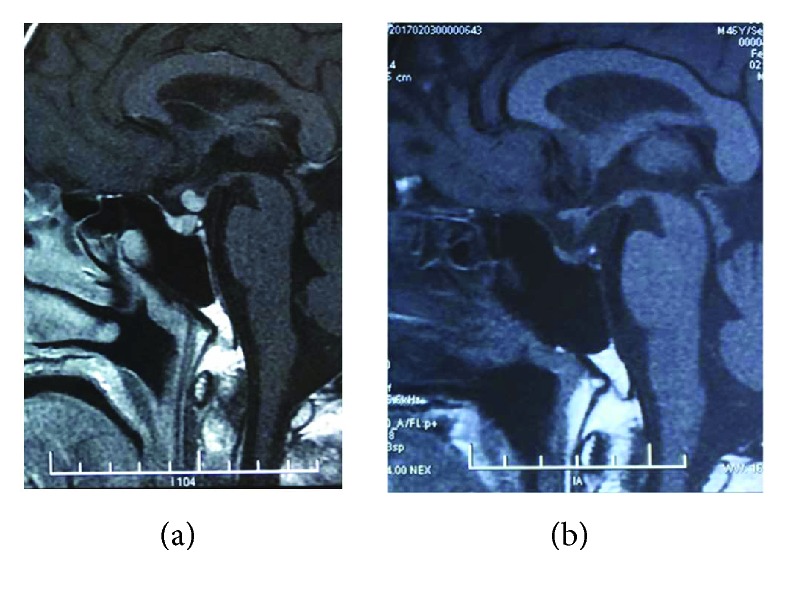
Pituitary MRI of patient 5 (a) before and (b) 1.2 months after treatment with glucocorticoids. The thickened pituitary stalk shrunk significantly.
